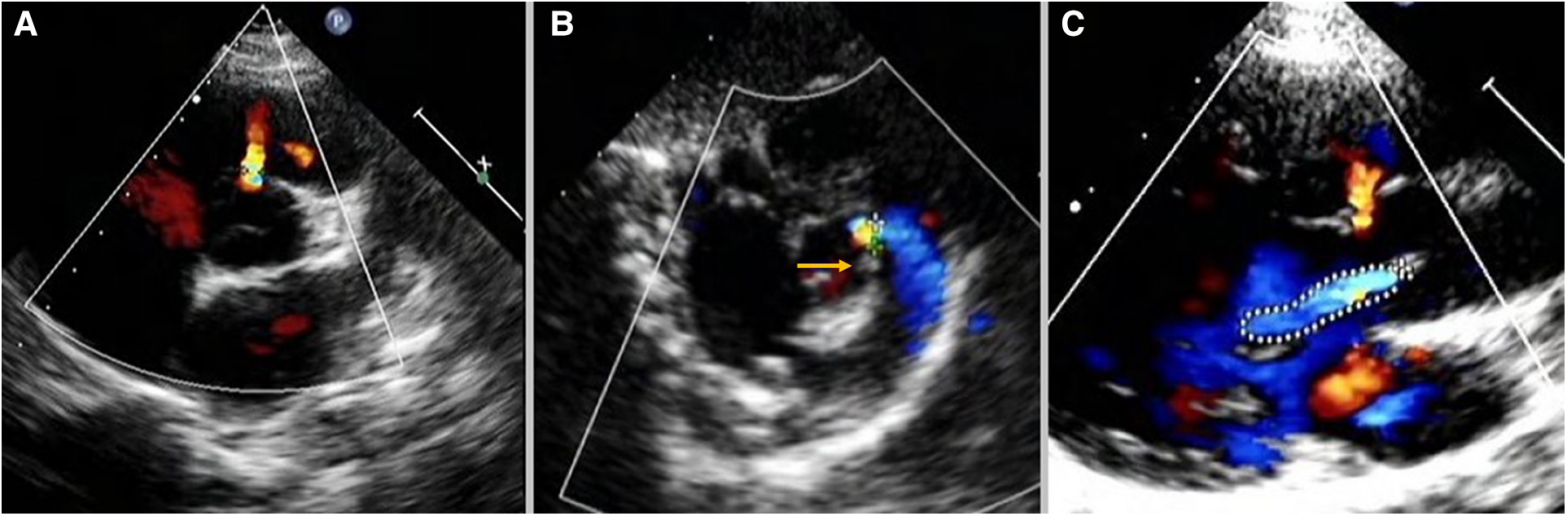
Intracristal VSD was diagnosed on TTE: (A) in parasternal short-axis sections, the defect was demonstrated at approximately 12-o’clock with the aortic valve seen face on. (B) The yellow arrow shows the presence of subpulmonary infundibulum precluded the diagnosis of doubly committed juxta-arterial VSD. (C) The defect was adjected to the right aortic valve leading to AVP and AR. TTE, transthoracic echocardiography; VSD, ventricular septal defect; AVP, aortic valve prolapse; AR, aortic regurgitation.
